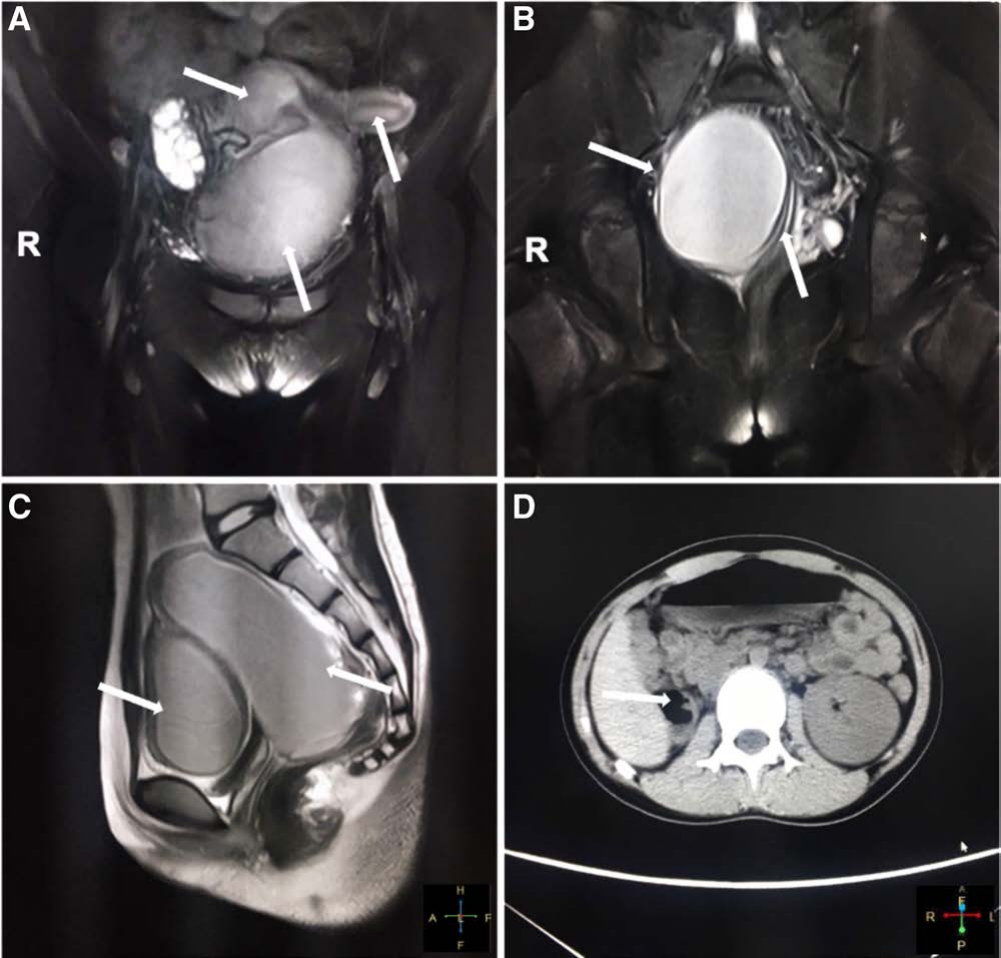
Magnetic resonance imaging and electron tomography findings of the patient. (A) Right uterus with increased hematoceles (upper right arrow), left uterus (upper left arrow), hematocolpos (lower arrow). (B) hematocolpos (upper right arrow). The left cervix was compressed into a narrow change due to hematocolpos (left arrow). (C) Right uterus with increased hematoceles (right arrow), hematocolpos (left arrow). (D) Non-visualization of the right kidney (right arrow).
